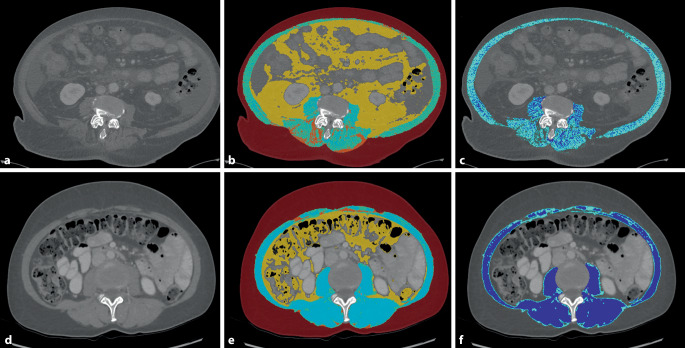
Body composition analysis at the L3/L4 lumbar level and tissue segmentation in two patients with pancreatic ductal adenocarcinoma. a–c Imaging of an 81-year-old male patient with cachexia showing low muscle attenuation and low muscle area as well as low subcutaneous and high visceral fat area. d–f Imaging of a 49-year-old female patient without cachexia showing high muscle attenuation and area as well as high subcutaneous and low intramuscular fat area. a, d CT source image. b, e CT image with body composition overlay (*light blue*: skeletal muscle, *yellow*: visceral fat, *maroon*: subcutaneous fat, *orange*: intermuscular fat). c, f CT image with skeletal muscle body compartment overlay (*dark blue*: lean muscle, *light blue*: intramuscular fat, *olive*: intermuscular fat)
